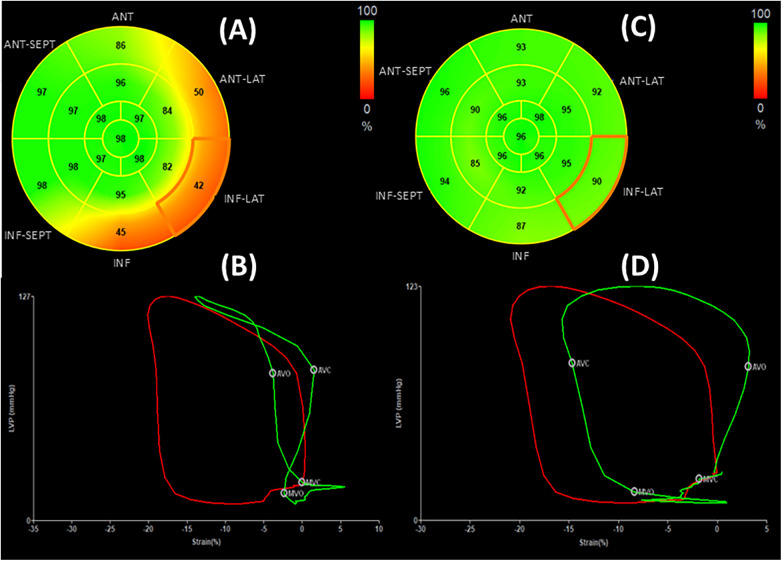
Segmental data before and after ablation of left inferolateral AP. Myocardial work efficiency, global LV pressure-strain loop (red) and segmental pressure-strain loop from the ventricular AP insertion site (green) before (A) and (B) and after (C) and (D) AP ablation. The area under the pressure-strain loop corresponds to the MWI. Normalization of both the segmental loops and segmental MWE at the AP insertion is seen after ablation. ANT, anterior; ANT-LAT, anterolateral; ANT-SEPT, anteroseptal; AP, accessory pathway; AVC, aortic valve closure; AVO, aortic valve opening; INF, inferior; INF-LAT, inferolateral; INF-SEPT, inferoseptal; LV, left ventricular; LVP, left ventricular pressure; MVC, mitral valve closure; MVO, mitral valve opening; MWE, myocardial work efficiency; MWI, myocardial work index. Figure 5 consists of screenshots from the EchoPAC platform (see the echocardiography section).
